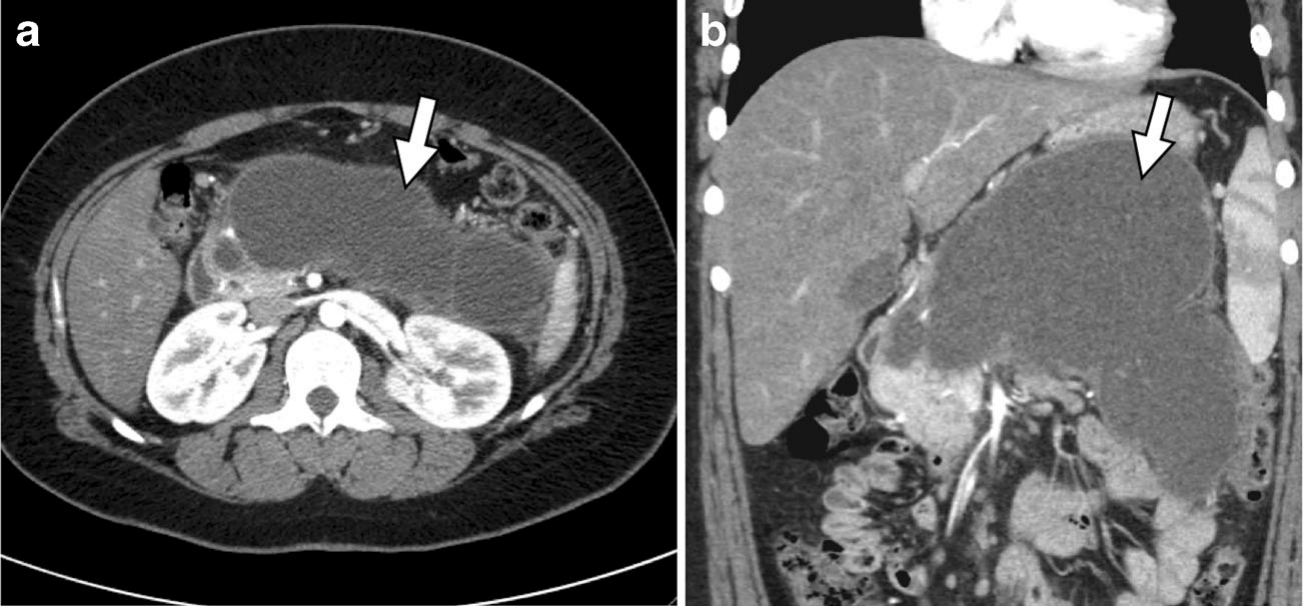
An 11-year-old male with acute lymphoblastic leukaemia on combination therapy that includes L-asparaginase. a, b Axial and coronal CT images, respectively, show “walled-off” necrosis (*arrows*) of the pancreas
